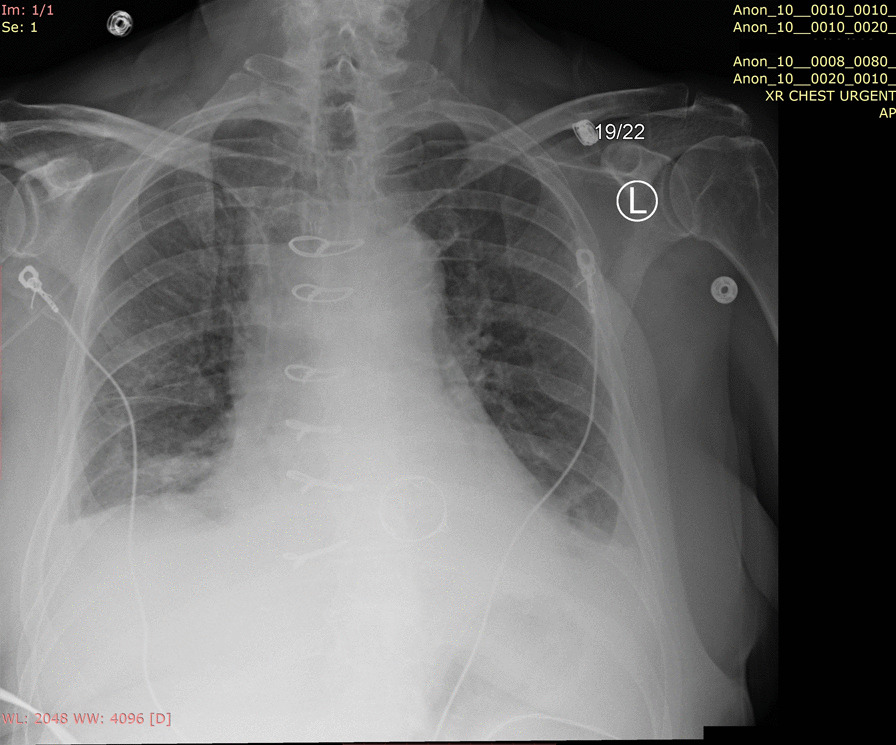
Chest X-ray revealed acute pulmonary edema
